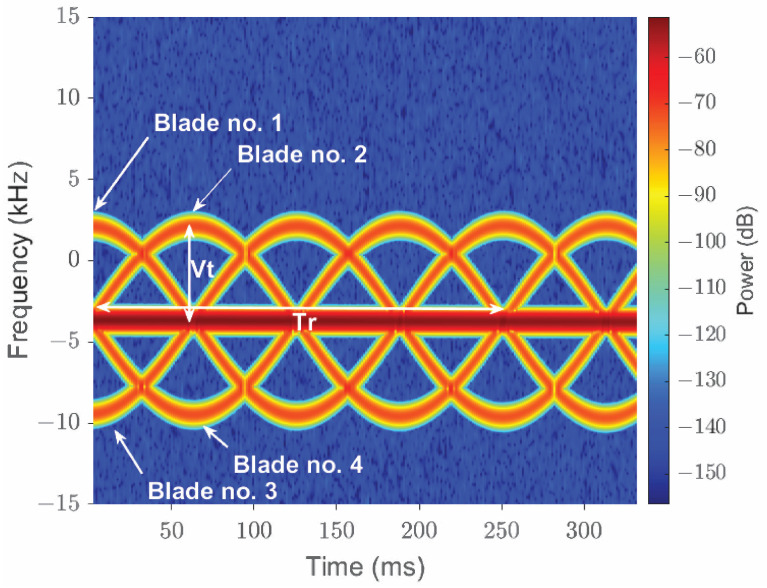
Micro-Doppler modulation due to (1) rotating blades and (2) a constant Doppler shift from the central rotating part of the helicopter (reprinted from [90]). The sinusoidal behavior is due to the rotation of the blades.
